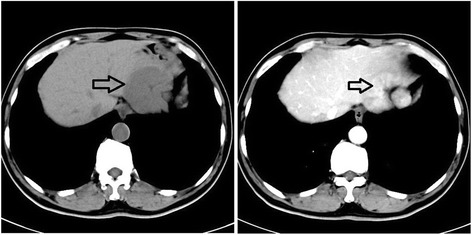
Computed tomography findings of the tumor in the liver. The tumor in the liver was located in the right lobe and was approximately 8.8 × 7.0 cm in size, as indicated by the arrows. The boundary is not clear and the density is uneven
